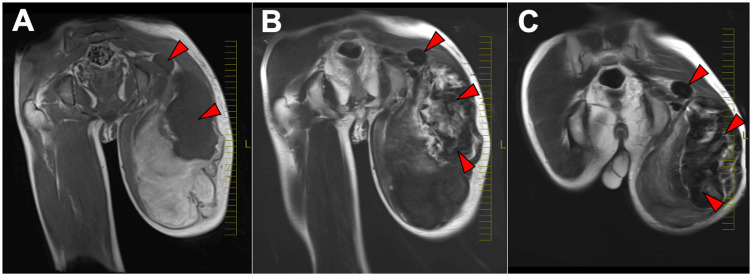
Coronal MRI obtained at presentation to the emergency room: (A) T1-weighted, (B) T2-weighted, and (C) contrast-enhanced T1-weighted images. A large heterogeneous mass with mixed high- and low-signal intensities extends from the left gluteal region to the posterior thigh. Signal-void areas (panels A-C, red arrows), consistent with a high-flow vascular component, are observed in the gluteal region.
